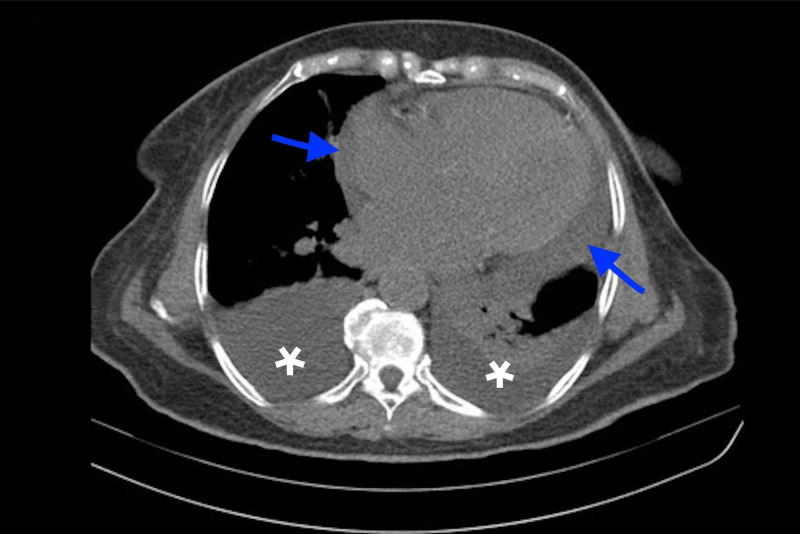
CT chest shows bilateral pleural effusions (white asterisks) and pericardial effusion (blue arrows).
